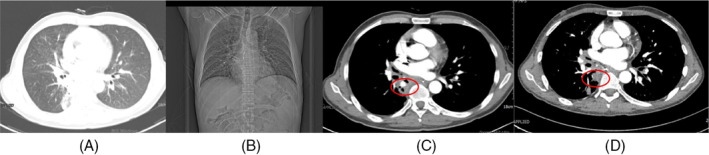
(A) Chest computed tomography (CT) lung window demonstrated a ‘mass’ before antifungal treatment; (B) the chest radiograph before antifungal treatment; (C) pseudo‐pulmonary artery aneurysm that appeared before antifungal treatment; (D) CT shows the disappearance of the pseudo‐pulmonary artery aneurysm after antifungal treatment.
